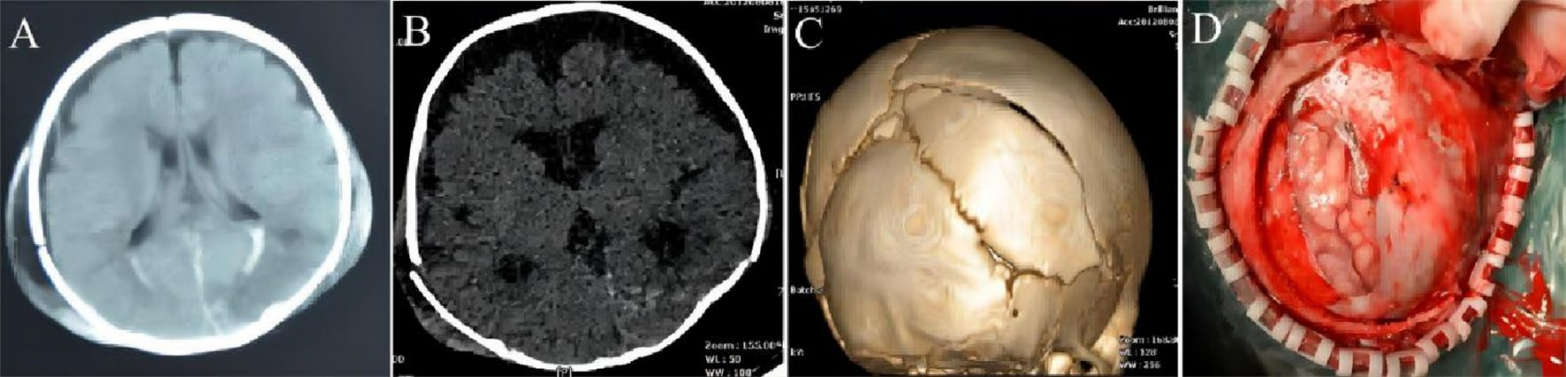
The imaging characteristics and intraoperative findings of Type II. (A) One day after the injury, CT indicated a linear fracture of the right parietal bone and subscalp hematoma and a large number of low-density shadows under the skin. (B) Nine days after the injury, CT showed local subscalp effusion, and bilateral subdural effusion. (C) 3D-CT showed a linear parietal bone fracture, fracture width greater than 4 mm. (D) The dural defect was obvious and not below the fracture line but below the bone flap, the brain tissue and the dural rim; after the adhesions were released, the subdural effusion was released.
